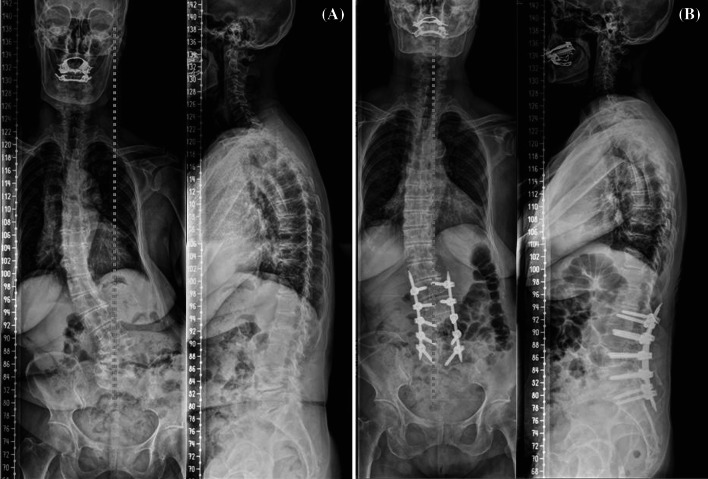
Multilevel MI-TLIF procedure. a Preoperative radiography; b postoperative radiography showing reduction of scoliotic curvature
